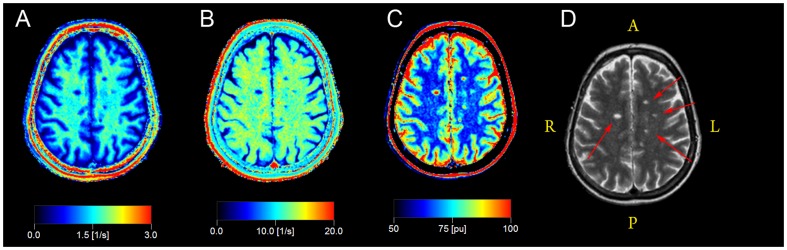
Sample qMRI data maps from a 45-years-old female MRI_pos_ MS patient. a) R1 map, b) R2 map, c) PD map, d) synthetic T2-weighted image is shown for reference. Several partially liquefied lesions are visible (red arrows in d).
